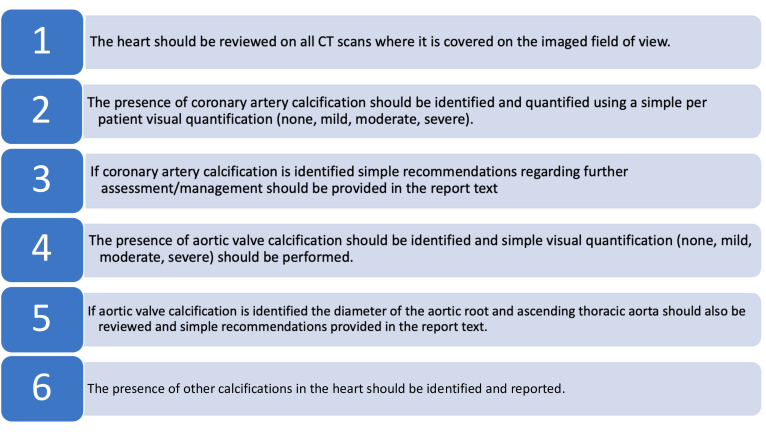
Recommendations for the assessment of cardiac calcification on routine thoracic CT
